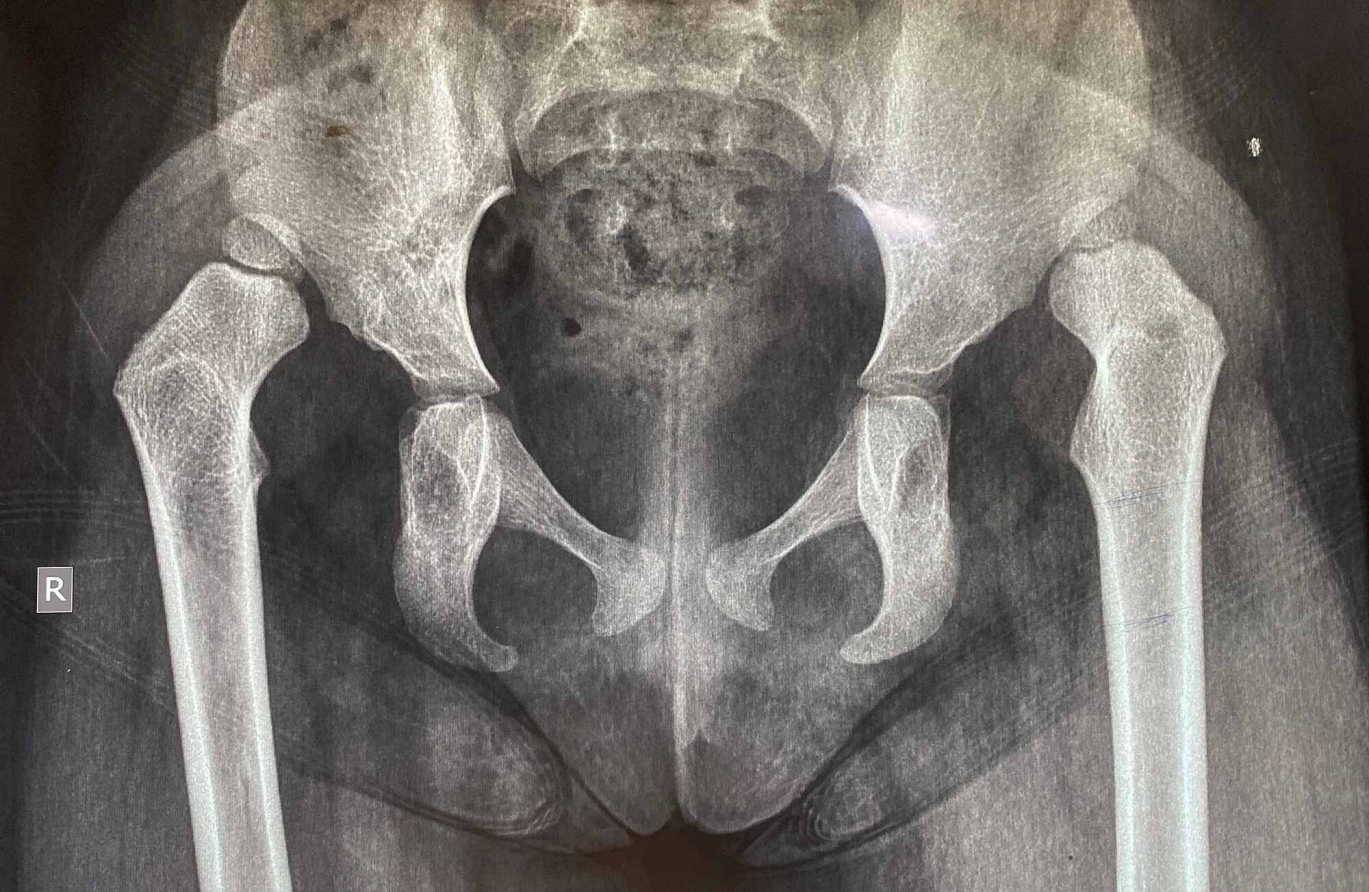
The X-ray of a three-year-old girl with bilateral DDH, Tonnis height of dislocation stage IV, both hips operated simultaneously DDH: developmental dysplastic hips
